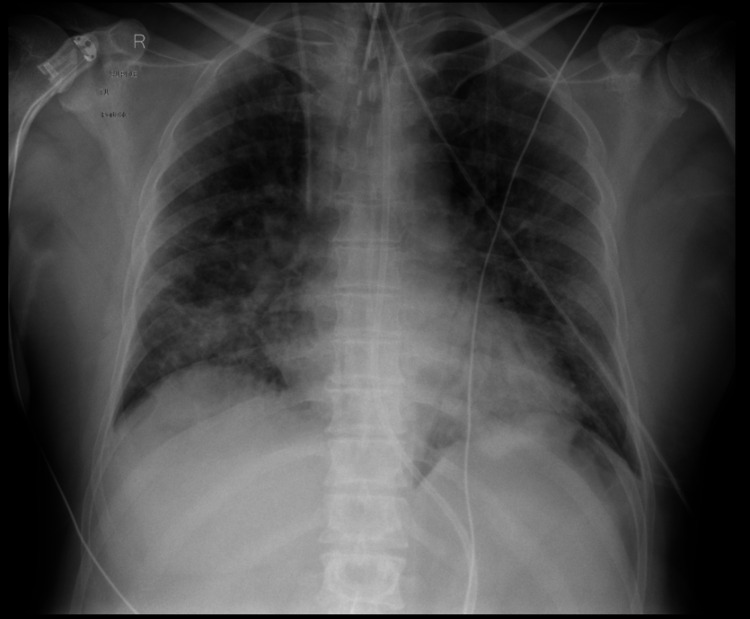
Chest radiograph Chest radiograph showing extensive bilateral pulmonary nodular infiltrates.
